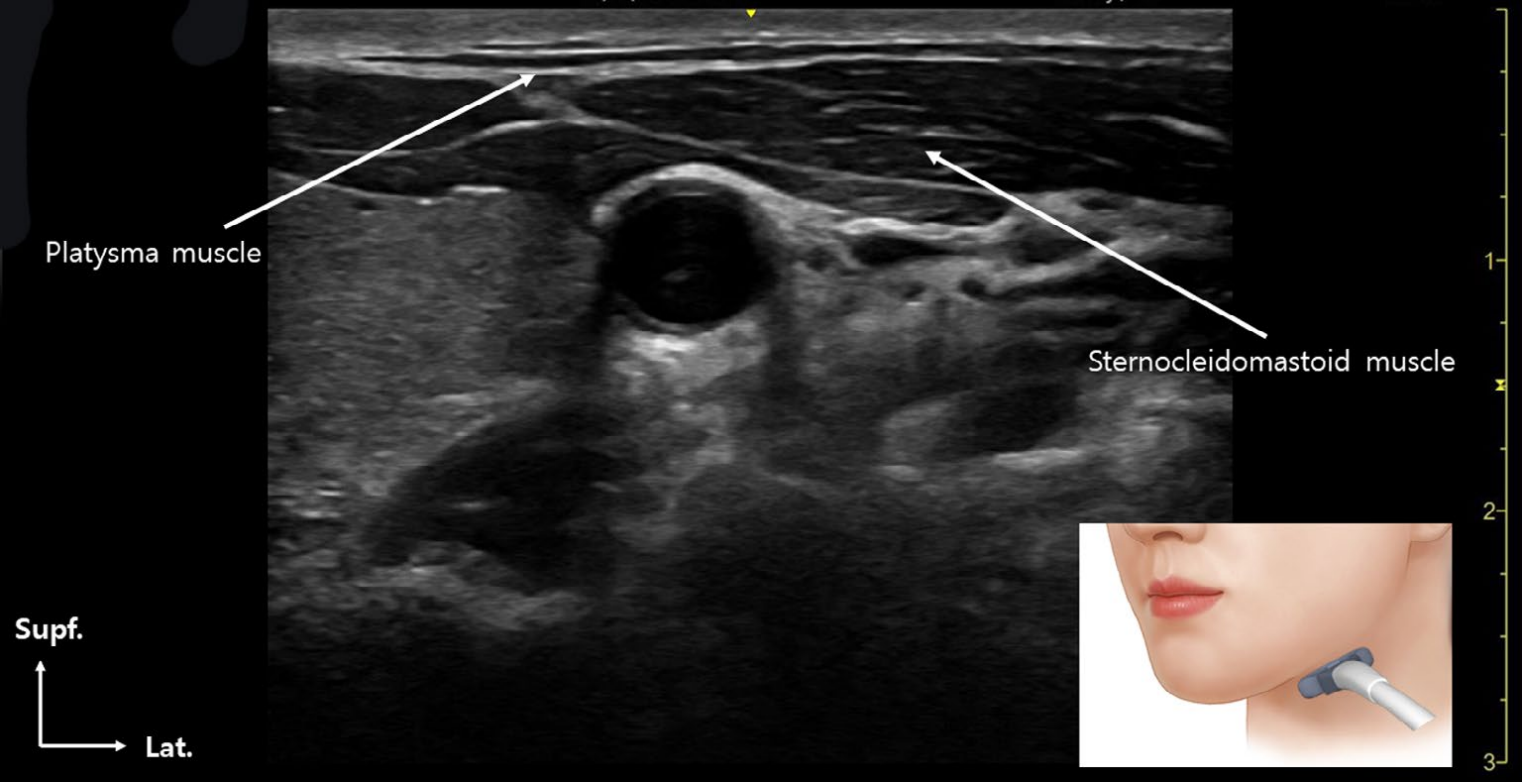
Platysma muscle observed under ultrasound guidance. This approach facilitates accurate botulinum toxin injection into the targeted muscle layer.
